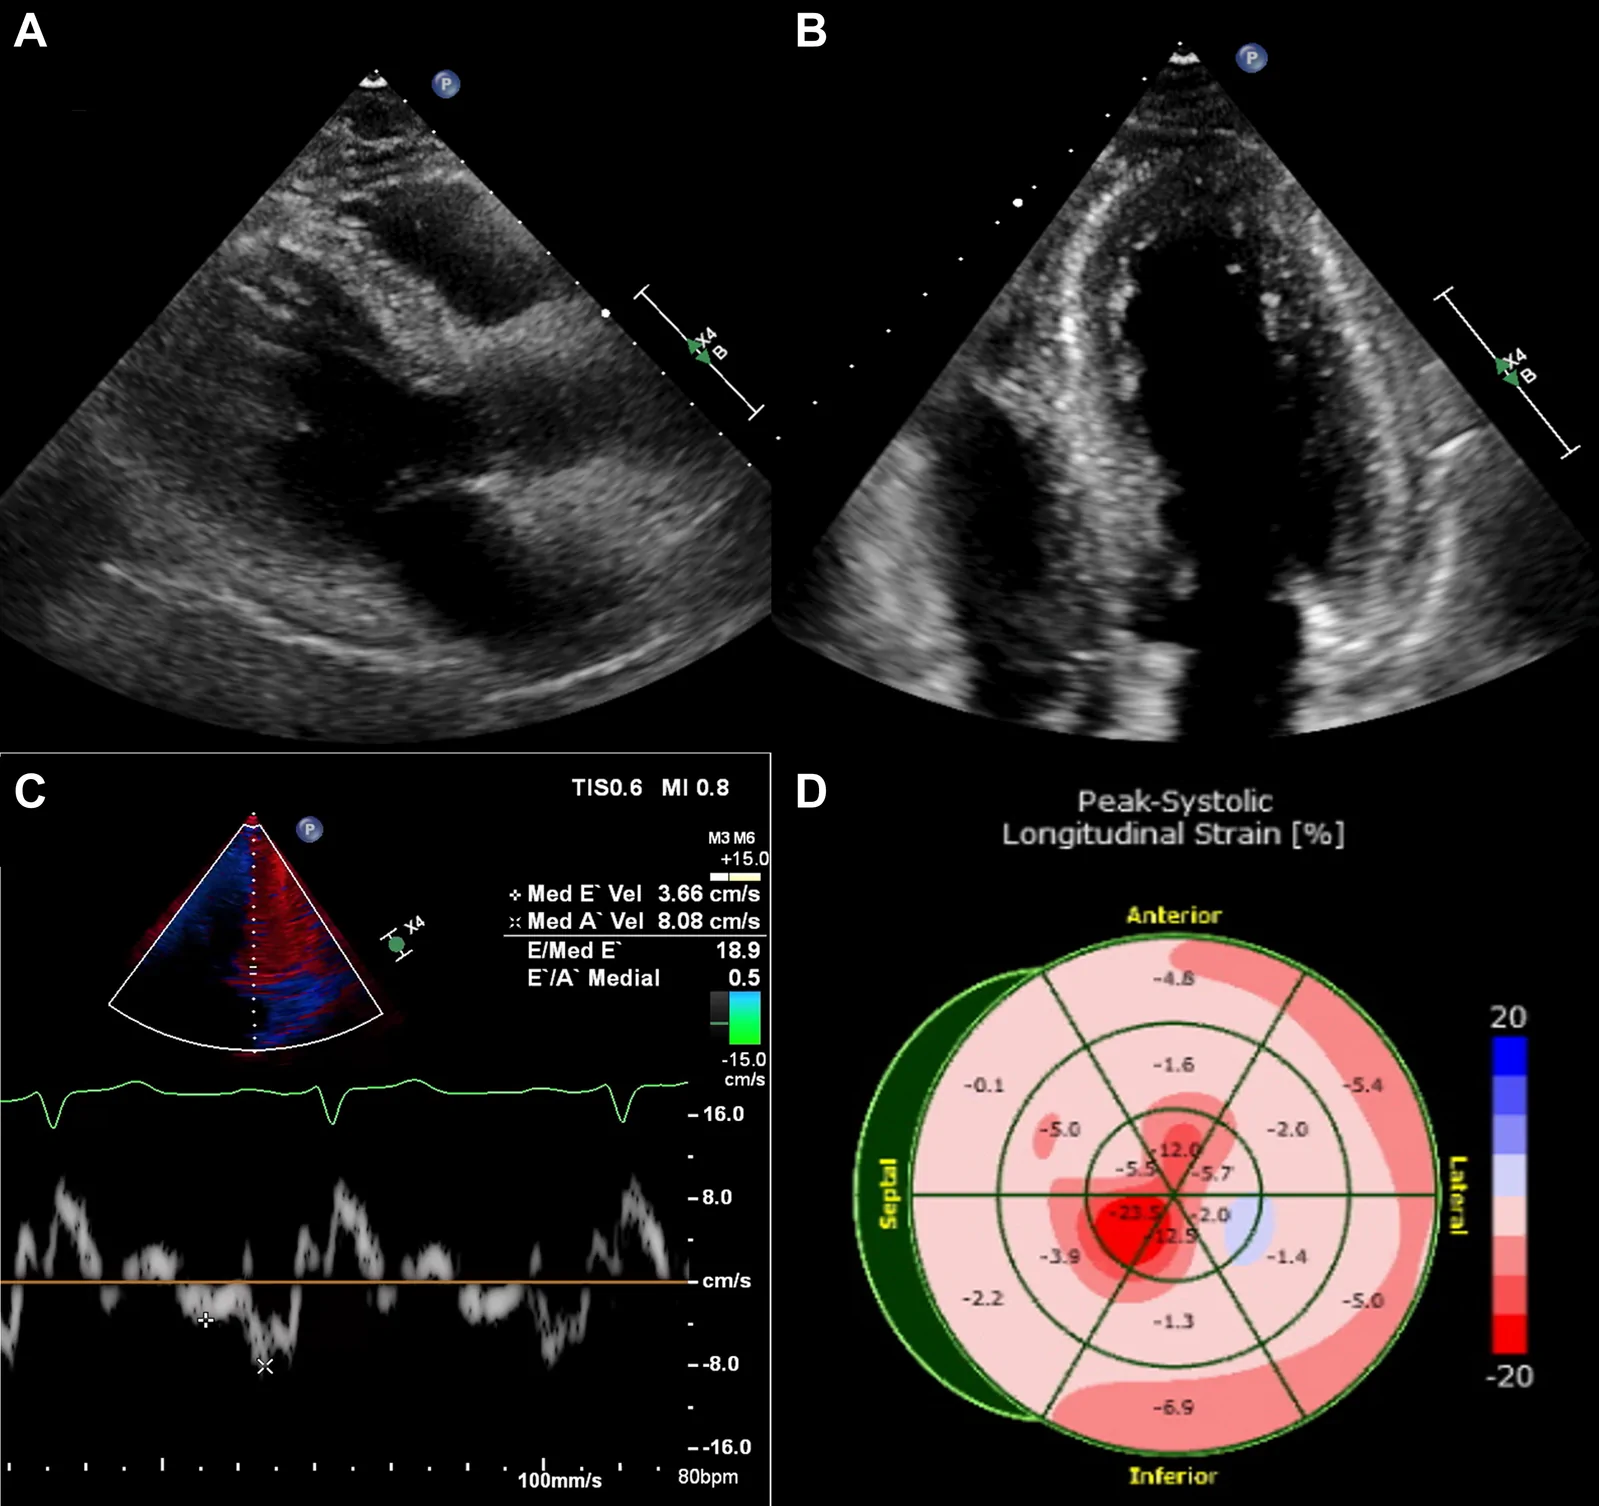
Echocardiography Echocardiography showed concentric left ventricular hypertrophy without relevant valvular disease (parasternal long-axis view) (A). Interventricular septum of 17 mm and inferolateral wall of 12 mm, resulting in an increased relative wall thickness of 0.63 and an indexed left ventricular mass of 123 g/m^2^ (apical 4-chamber view) (B). Diastolic dysfunction was present with an E/A ratio of 0.8, medial e' of 3.7 cm/s, and an elevated E/e' ratio of 18.9 (C). Strain imaging showed reduced systolic longitudinal strain (−5.2%) with apical sparing (D).
